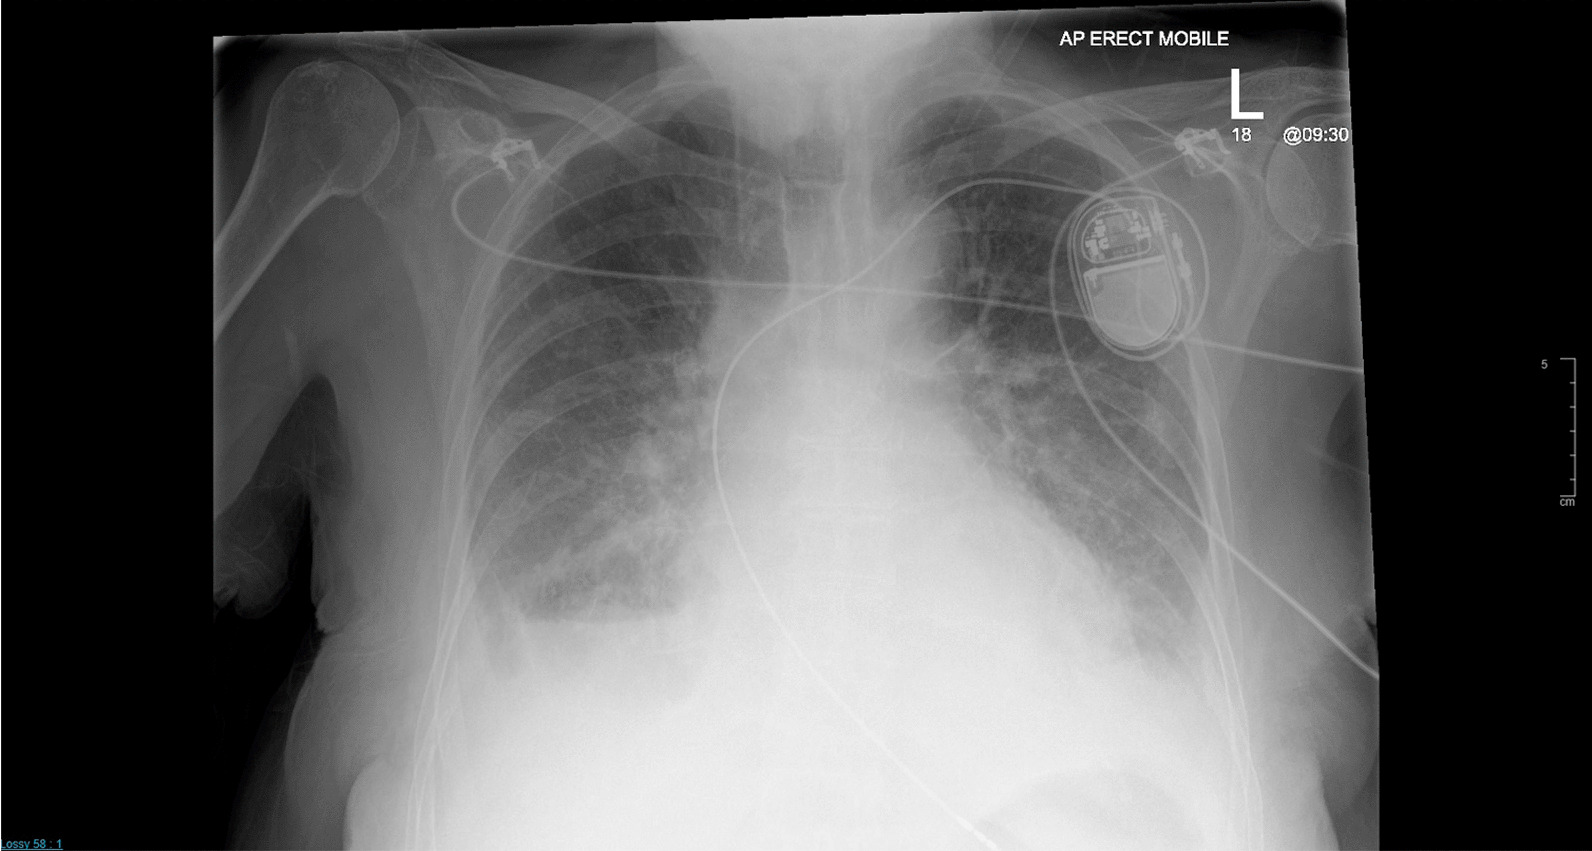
Chest X-ray taken in posteroanterior view following readmission with shortness of breath demonstrates cardiomegaly and features keeping in with heart failure
